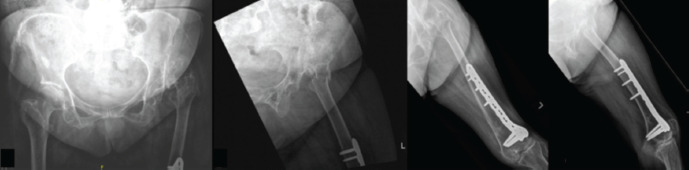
Pre-operative radiographs showed a left hip intertrochanteric fracture and concurrent left dysplastic hip. The left femur radiographs showed left distal femoral plate proximally was more anterolateral due to altered femoral anatomy.
